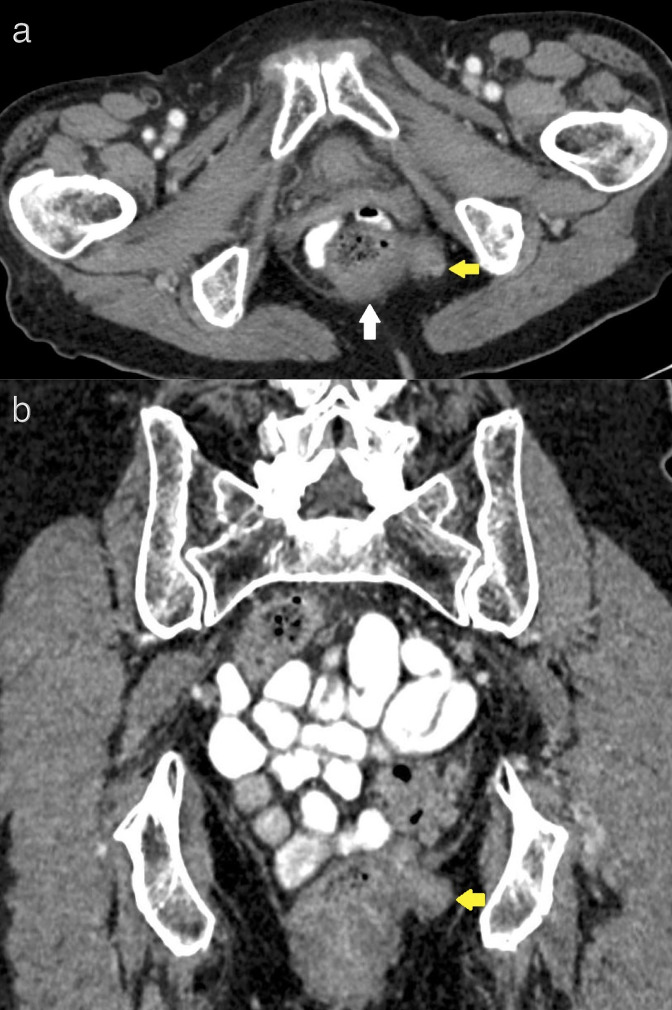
Axial (a) and coronal (b) contrast-enhanced, portal venous phase CT images through the pelvis at the level of the ischial fossae demonstrate eccentrically thickened left posterolateral rectal wall (white arrow) with an exophytic component (yellow arrow) breaching the meso-rectal fascia and extending into the left ischiorectal fossa at 4 o‘clock position.
